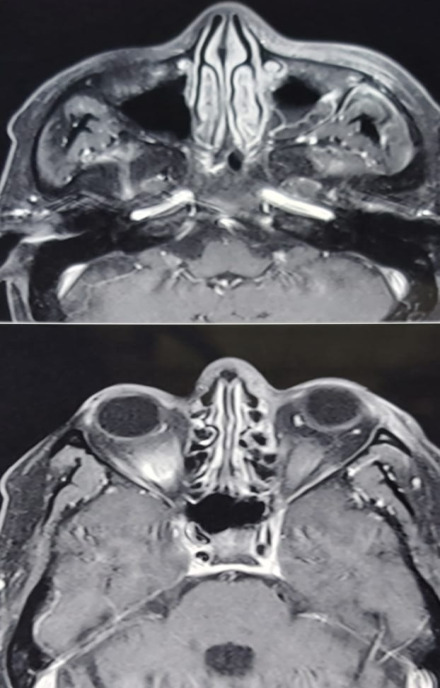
Axial section of MRI brain, PNS and orbits showing moderate mucosal thickening of the left maxillary sinus and both the ethmoidal sinuses. Thickened left optic nerve sheath can also be seen
